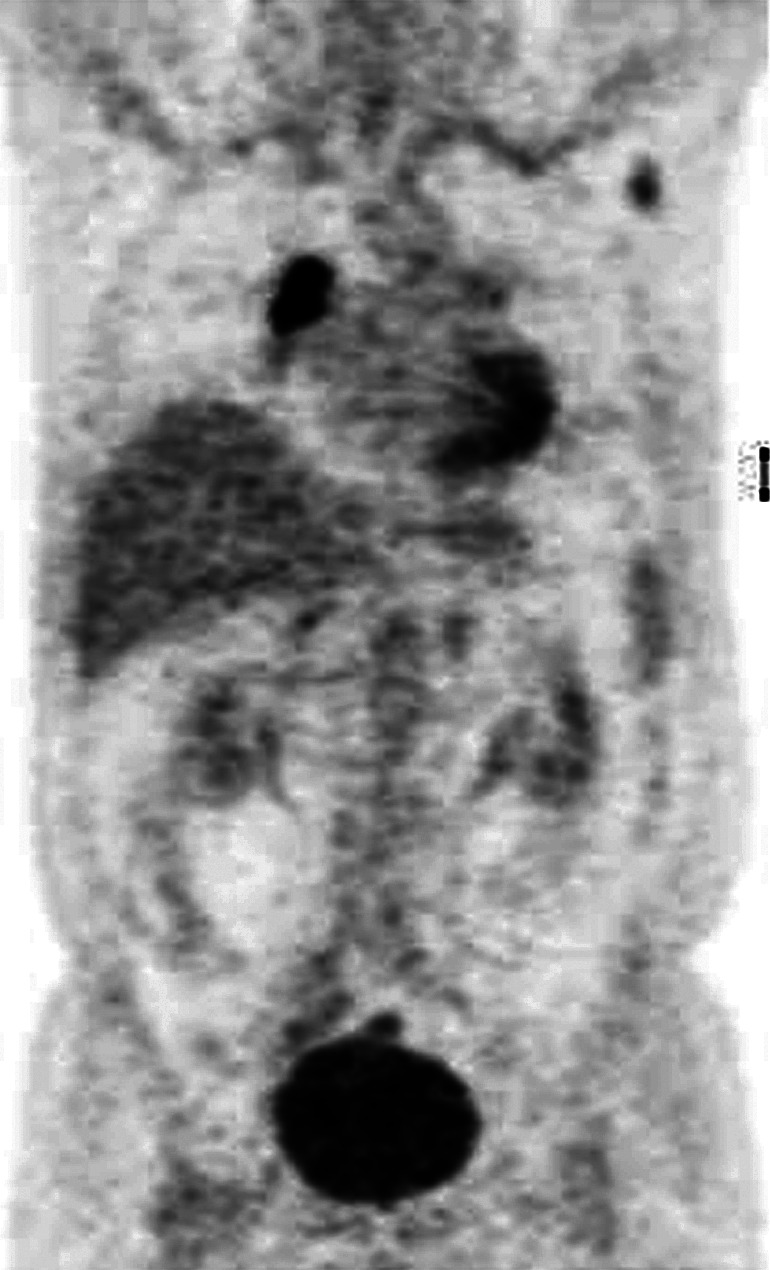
[^18^F]FDG-PET/CT image of a 74-year-old male patient, 4 months after the initial surgery. The [^18^F]FDG-PET/CT performed to assess for suspected recurrence showed increased uptake of [^18^F]FDG in the left axillary lymph nodes (SUVmax = 4.2), right hilar lymph nodes (SUVmax = 17.5) and lymph nodes in the anterior mediastinum, behind the sternum (SUVmax = 3.3). The previously performed CT had not correctly assessed the mediastinal lymph nodes due to their small size. FDG-PET/CT, fluorodeoxyglucose-positron emission tomography/computed tomography; SUVmax, maximum standardized uptake value.
